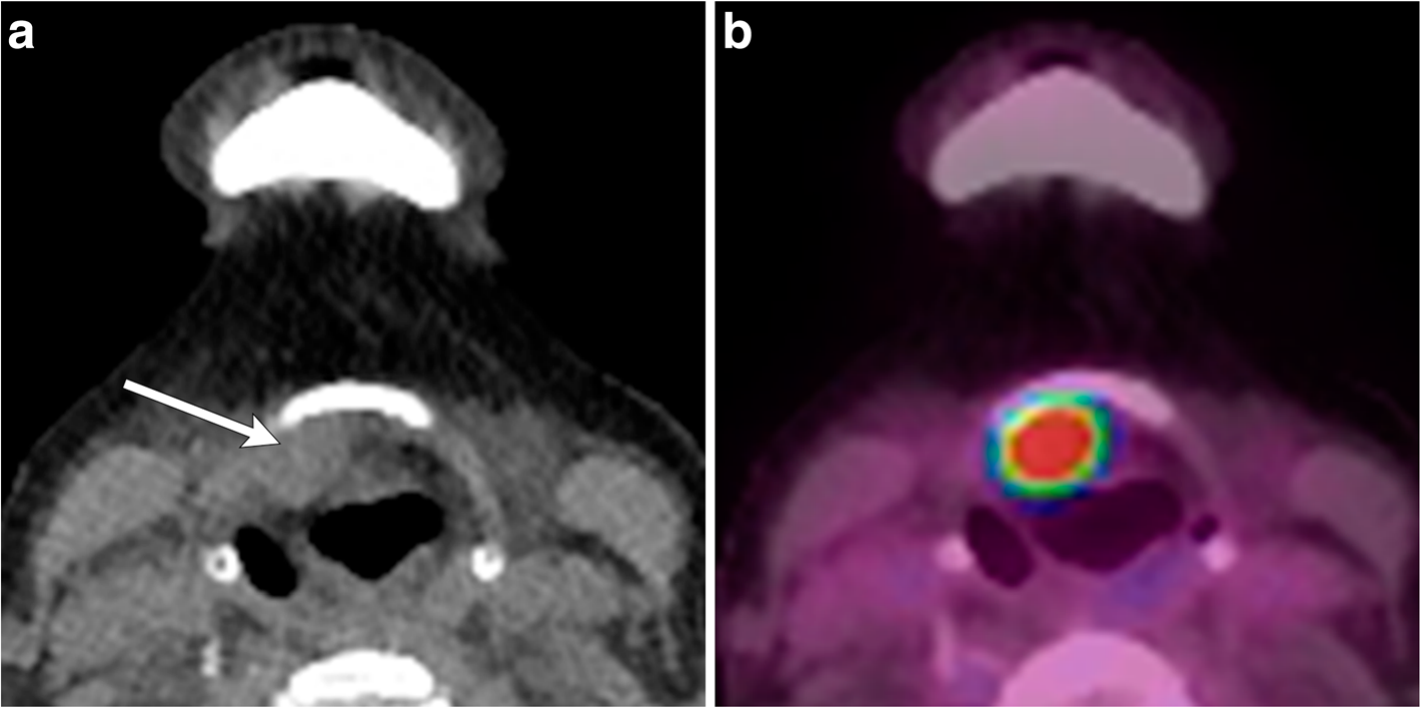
Malignancy of thyroglossal duct remnant. a Axial non-contrast CT image demonstrates a soft tissue mass adjacent to the posterior margin of the hyoid bone (arrow). b Axial fused PET/CT image demonstrates FDG avidity in this lesion. Pathology proven papillary carcinoma
